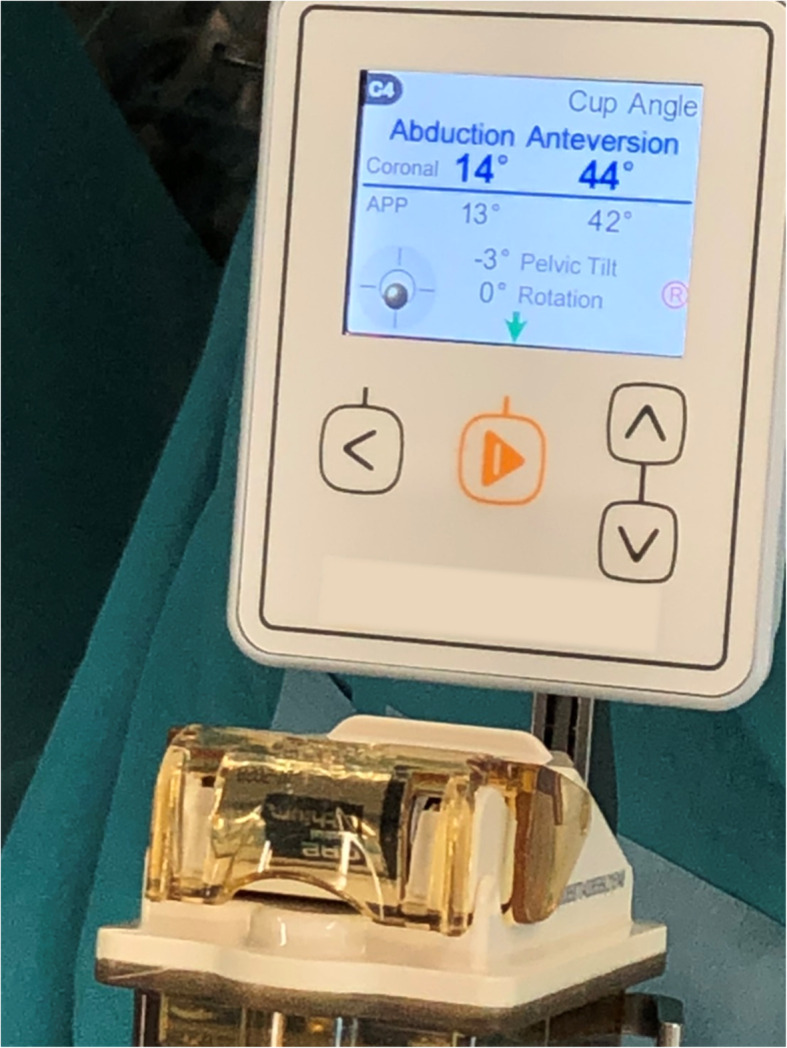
On the screen of the main unit, inclination and anteversion are displayed in radiographic values during cup insertion and after cup placement. Pelvic tilt and rotation are displayed at any given point in time
